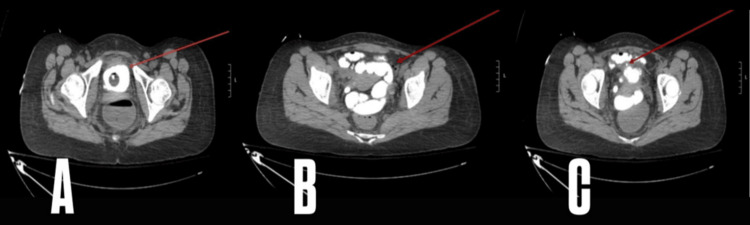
CT urography Figure 2A shows the CT urography demonstrating the contrast-enhanced bladder (arrow), while Figure 2B demonstrates contrast-enhanced colonic air-fluid levels (arrow), and Figure 2C shows the location of the fistula (arrow). CT, Computed tomography
